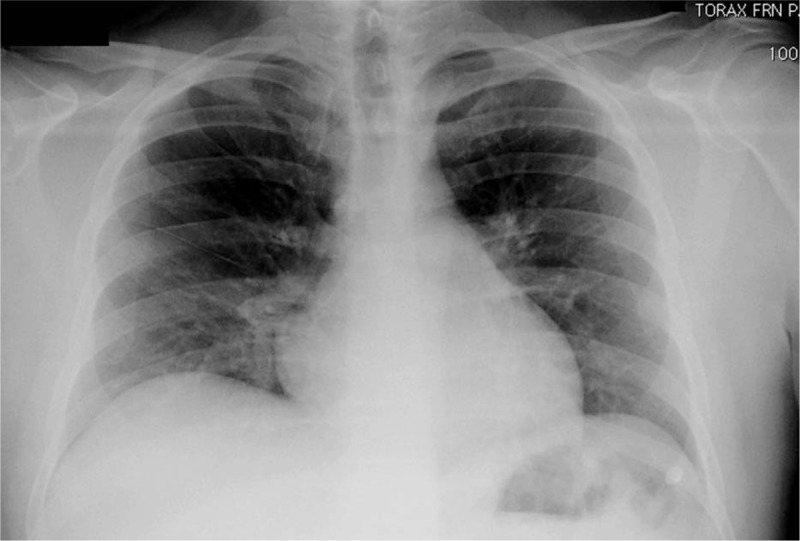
Chest radiograph on postoperative day 3.
